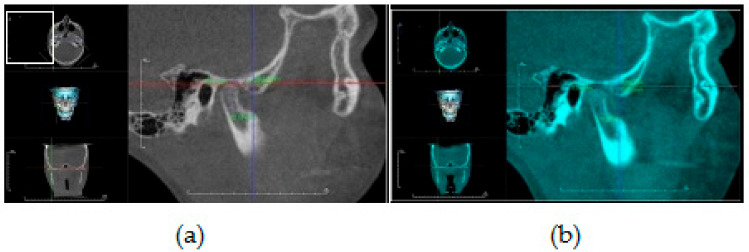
CBCT sagittal generated view showing assessment of condylar head position relative to VP (blue line) and FHP (red line): (a) before (T1) TB therapy; (b) after TB therapy (T2).
